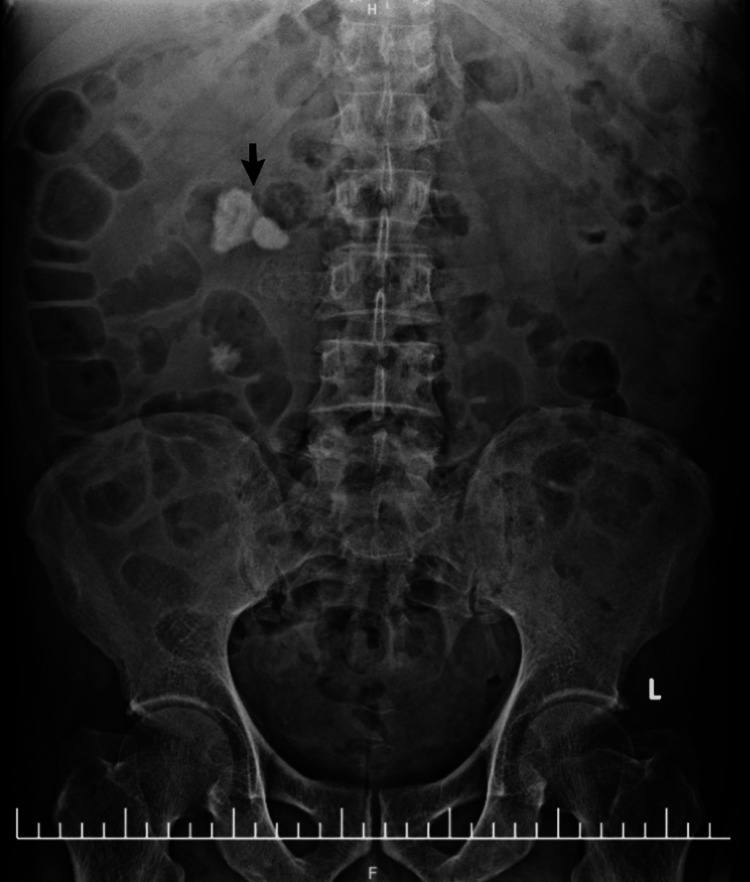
Plain radiograph of the kidneys, ureters, and bladder (KUB). Plain abdominal radiograph clearly demonstrating a large, prominent radio-opaque calculus (arrow) located in the anatomical region of the right renal pelvis and pelviureteric junction.
